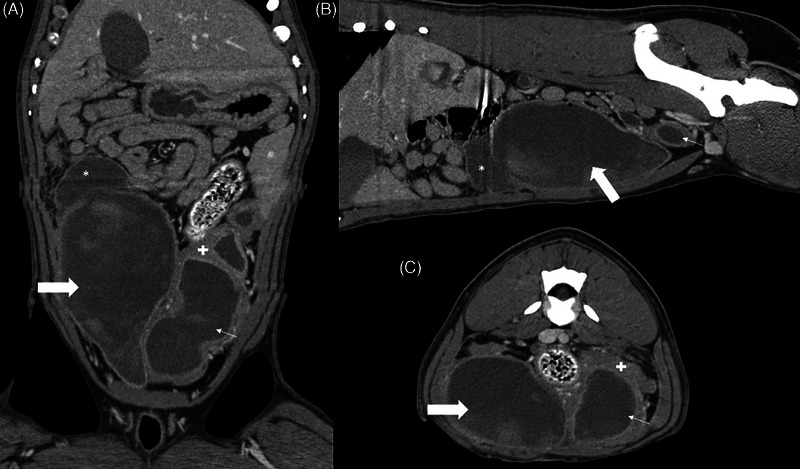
Dorsal (A), sagittal (B), and transverse (C) CT images postcontrast medium administration centered at the level of the urinary bladder and uterus. At the apex of the bladder is a crescent‐shaped‐fluid‐filled cavity (*), increased in size from the ultrasound examination (Figure 1) which communicates with the urinary bladder. The bladder (thick arrow) contains some relatively hyperattenuating material, similar in character to the left uterine horn (thin arrow). The uterine wall is moderately thick (+).
